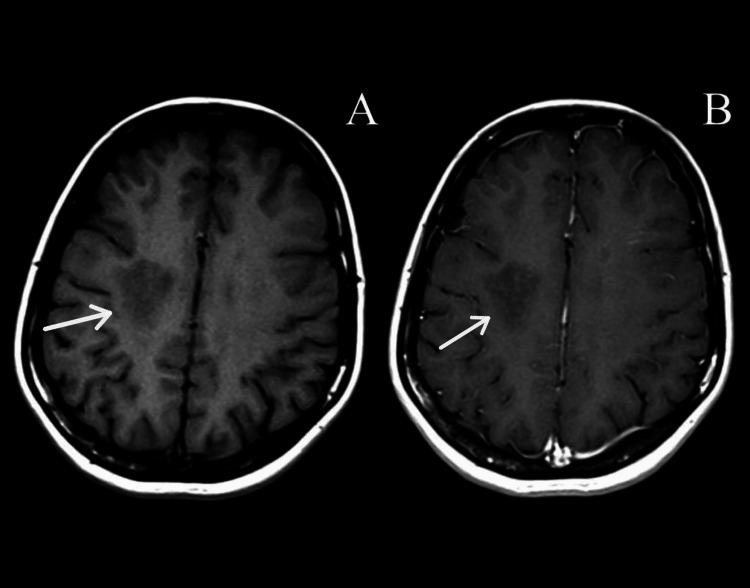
Brain MRI (A) axial T1 and (B) axial T1 post-contrast show no enhancement of the lesion in deep white matter of the right frontal lobe.
